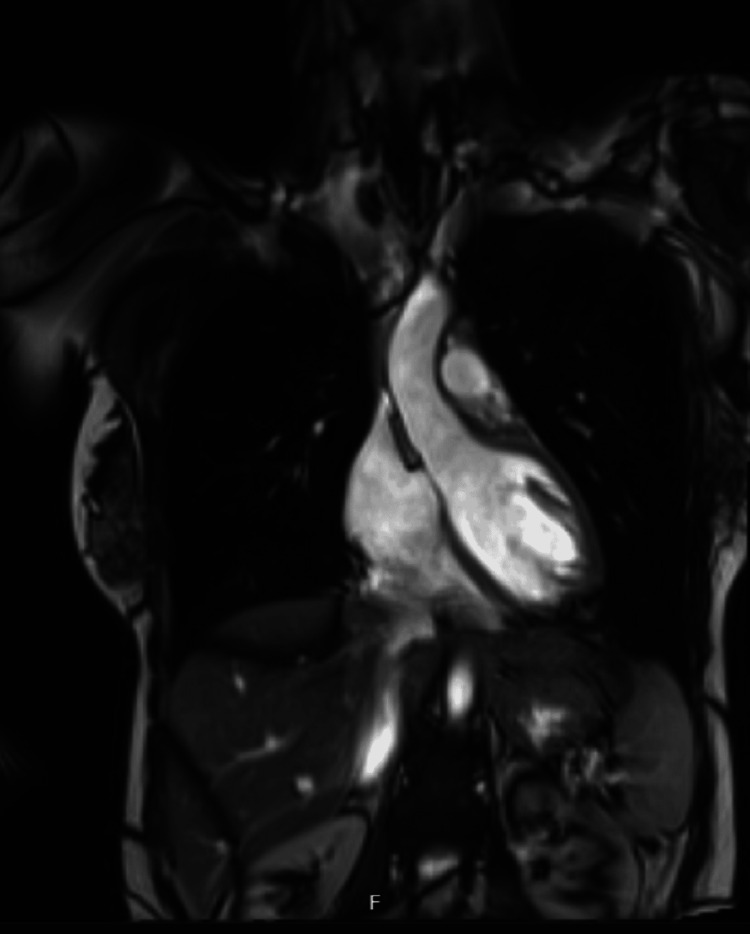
Cardiac MRI (coronal section): normal LVOT MRI: magnetic resonance imaging, LVOT: left ventricular outflow tract
